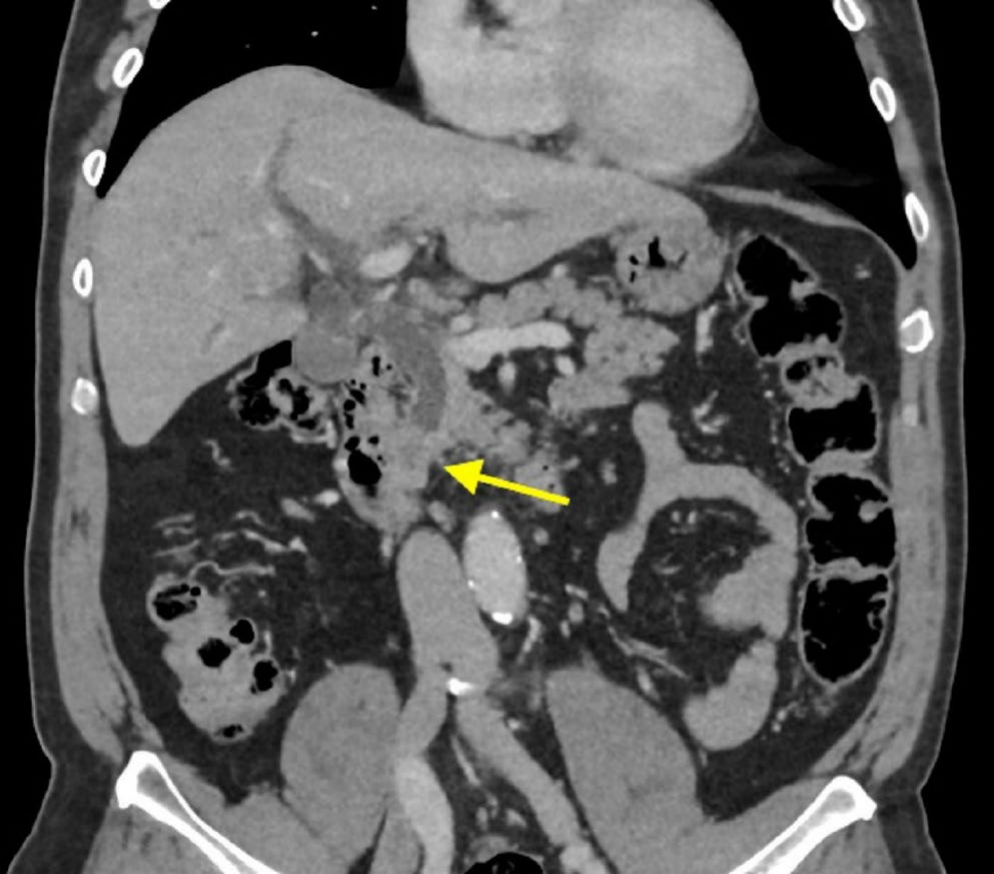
Coronal portal venous phase CT showing abrupt truncation of the distal CBD with 2.4cm stricture/intraluminal soft tissue which extends to the level of the ampulla, as indicated by the arrow. Associated moderate upstream intra and extrahepatic biliary tree dilatation is present
